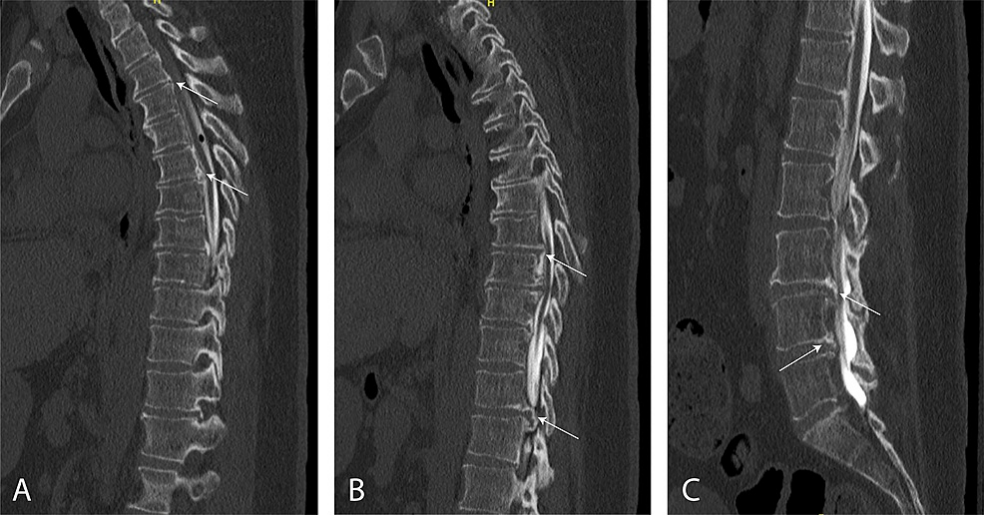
Sagittal CT myelogram demonstrating OPLL in the thoracic (A, B) and lumbosacral (C) spine from T2-5, T6-10, and L1-S1. The arrows indicate few areas of ossification visible on the image slice. CT, computed tomography; OPLL, ossification of the posterior longitudinal ligament
